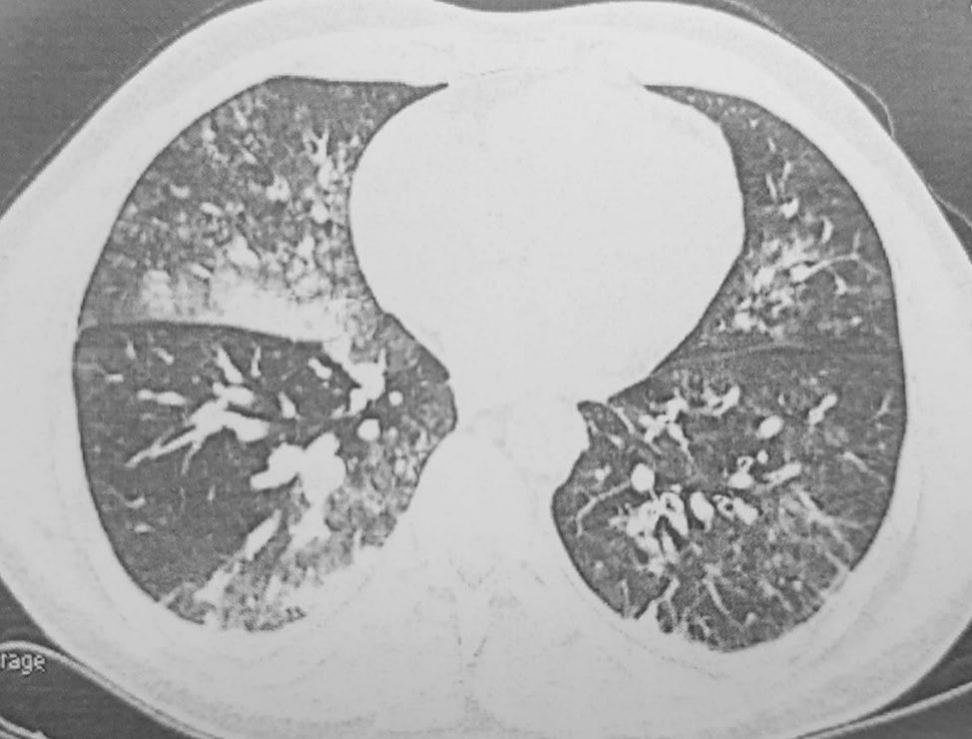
Ground glass opacities of both lungs in computed tomography scans
